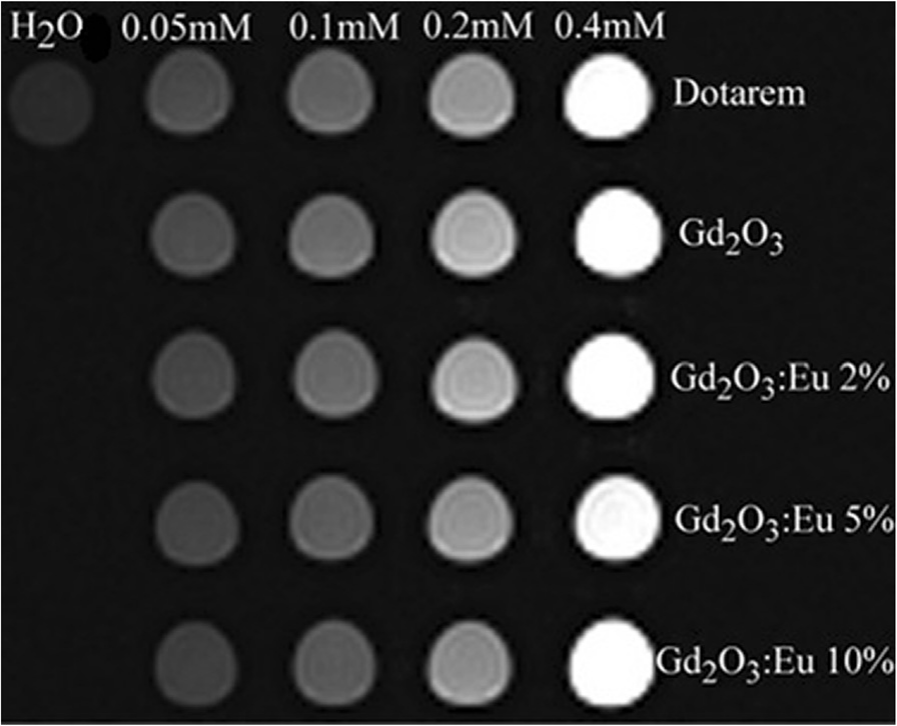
MRI of different concentrations (0.05 to 0.4 mM) of Gd_2_O_3_ and Gd_2_O_3_:Eu 2% to 10% compared to the same concentrations of commercial contrast agent (Dotarem).
